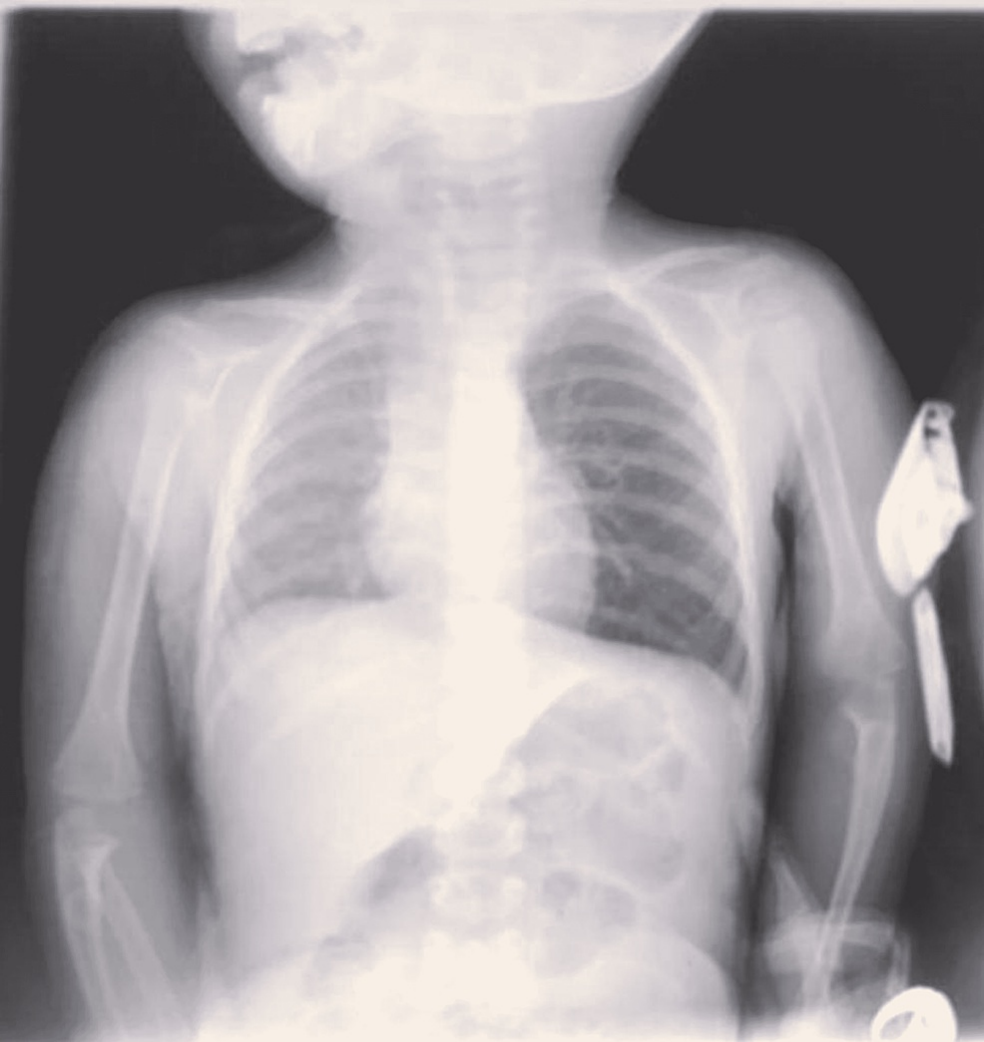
Pre-operative plain chest x-ray showed left lung hyperinflation.
